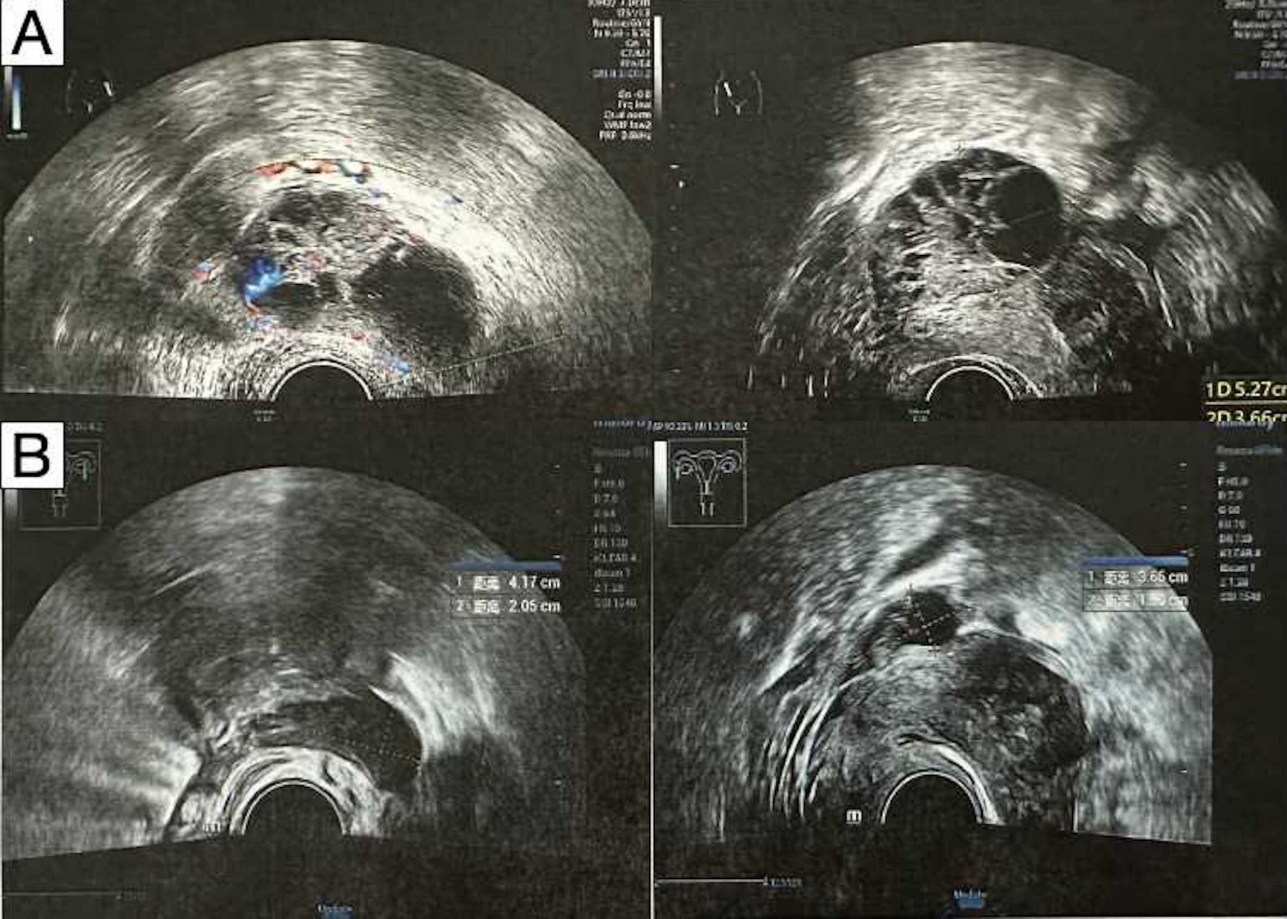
Transabdominal ultrasonography demonstrated bilateral adnexal masses. (A) Before neoadjuvant therapy. (B) After neoadjuvant therapy.
